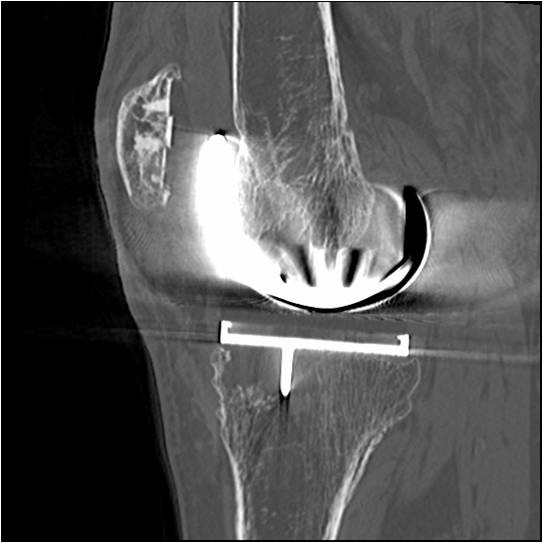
Sagittal CT demonstrating the lytic area in the antero-medial aspect of the tibia.
